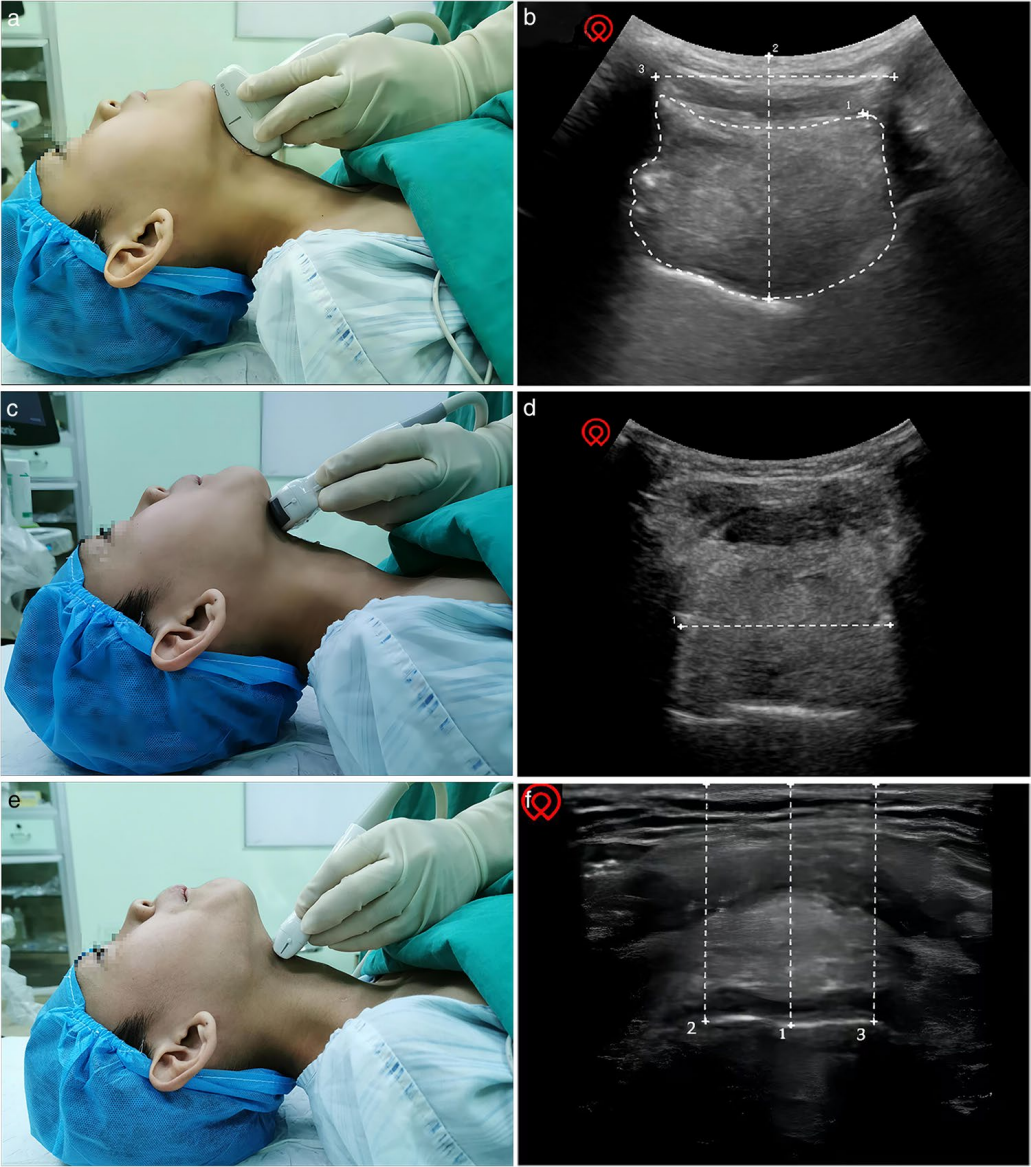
Positioning of the ultrasound probe and sonographic images of the submandibular area. a The low-frequency probe was placed under the chin in the midsagittal plane. b White dotted area: (1) tongue cross-sectional area (TCSA); (2) tongue thickness; (3) hyomental distance in the extended position (HMDE). c The low-frequency probe was placed transversely under the chin to obtain an entire tongue contour. d White dotted area: tongue width. e The high-frequency probe was placed transversely at the level of thyrohyoid membrane. f White dotted area: the vertical distances from the submental skin to the midpoint of the epiglottis and to the left and right extremities of the epiglottis, the mean of them was the distance from skin to epiglottis (DSE)
